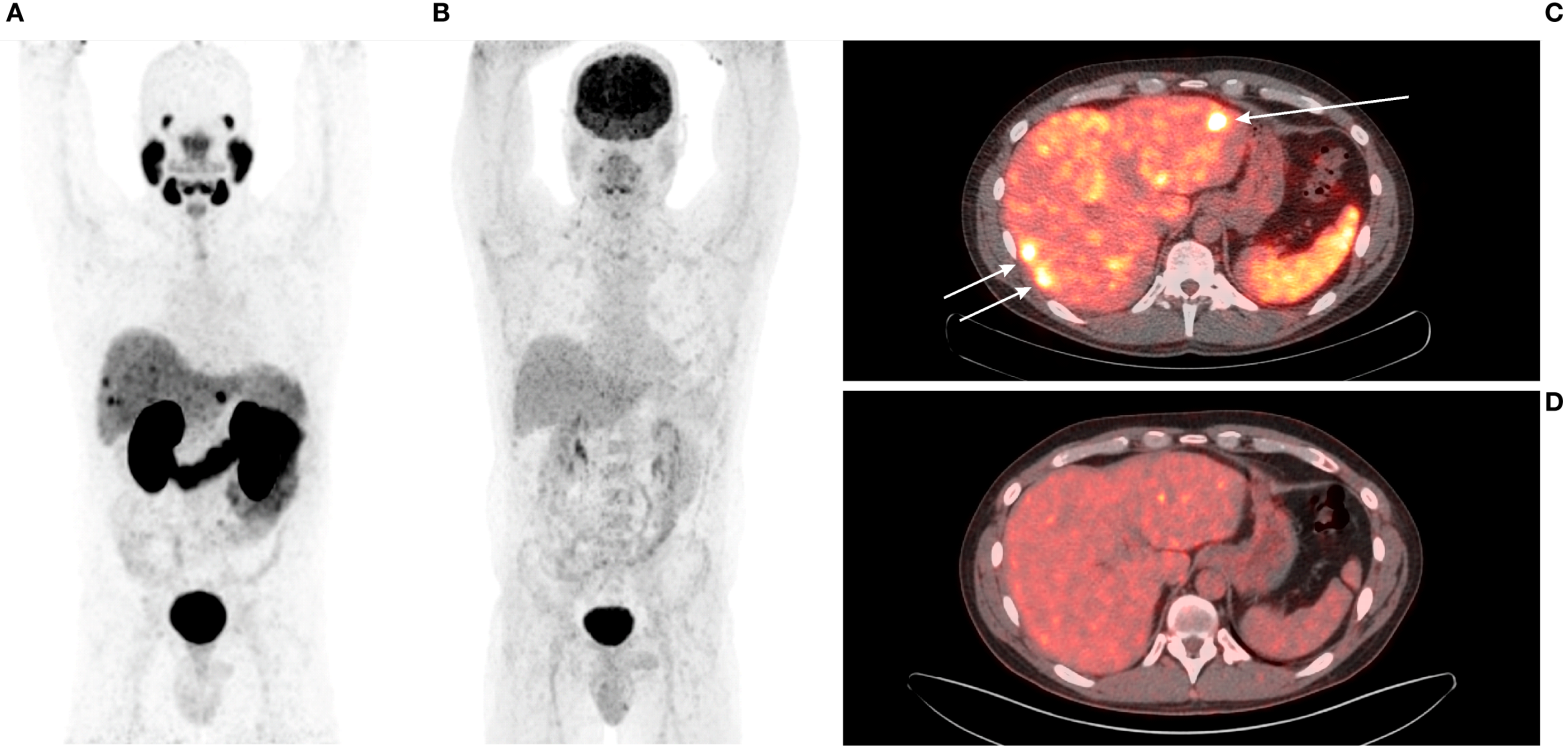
(A) 42-year-old male with medullary thyroid carcinoma and liver metastases. (A) [^68^Ga]Ga-PSMA-11 PET MIP. (B) [^18^F]FDG PET MIP. (C) Transaxial view of the fused [^68^Ga]Ga-PSMA-11 PET/CT scan (white arrows). (D) Transaxial view of the fused [^18^F]FDG PET/CT scan. Liver metastases are avid on [^68^Ga]Ga-PSMA-11 PET (mean SUV_max_ 9.25; mean TBR_Liver_ 2.82) and not avid on [^18^F]FDG PET (mean SUV_max_ 3.85; mean TBR_Liver_ 1.61). All metastases were undetectable on non-contrast CT (not shown in the Figure).
